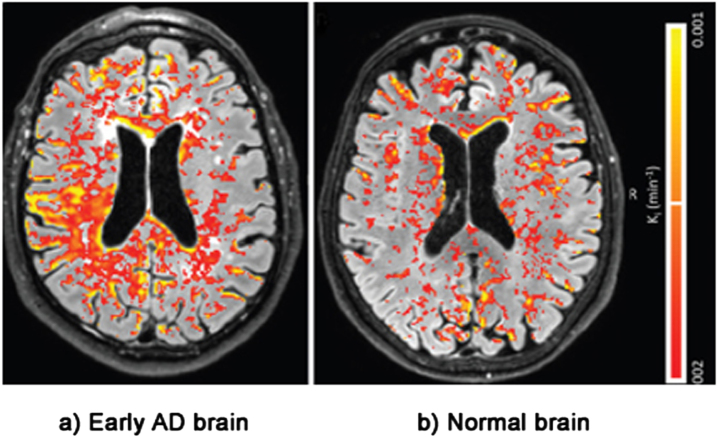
Evidence of BBB damage in the AD brain: (a) extensive leakage of gadobutrol (an MRI contrasting agent) through a damaged BBB in brains of patients with early signs of AD; (b) less extensive leakage of the agent in brains of normal patients. Used with permission of The Radiological Society of North America, from van de Haar HJ, Burgmans S, Jansen JFA, van Osch MJP, van Buchem MA, Muller M, Hofman PAM, Verhey FRJ, Backes WH, Blood-brain barrier leakage in patients with early Alzheimer disease, *Radiology* (2016) 281, 527–535.
